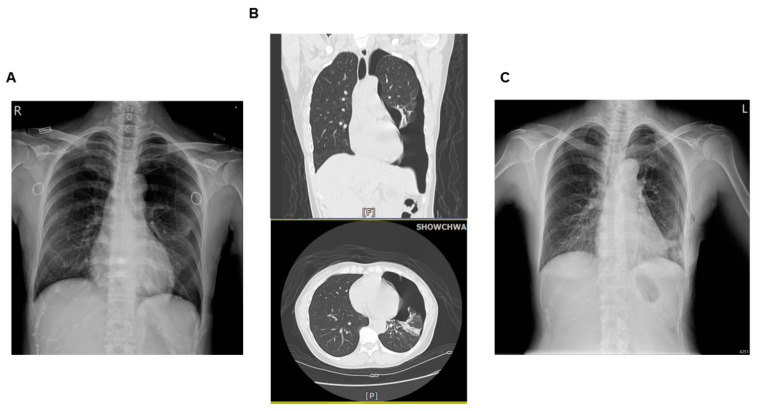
(A) Chest X-ray (CXR) and (B) computed tomography (CT) after acupuncture treatment (F: Front; P: Posterior). (C) CXR taken when the patient was discharged from the hospital.
